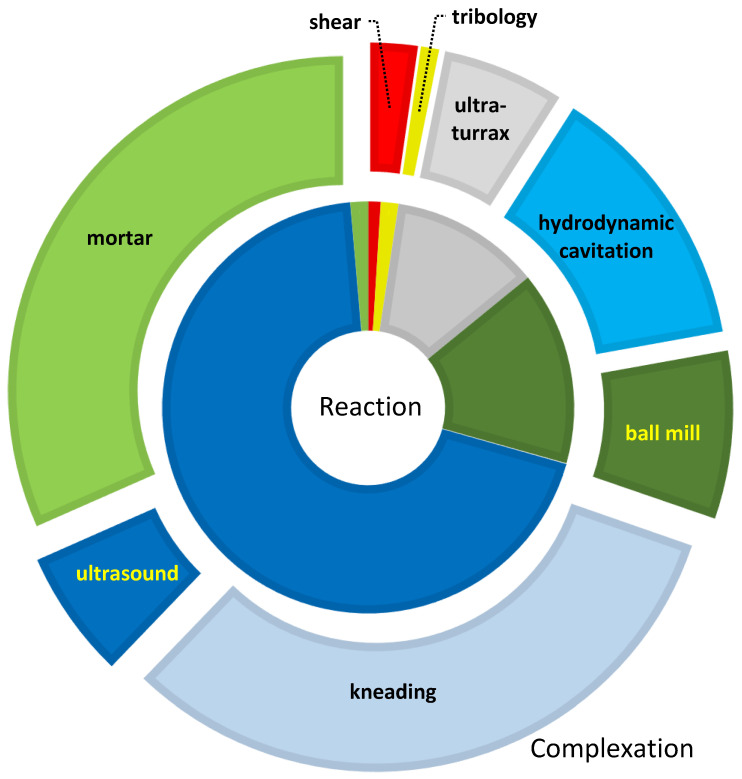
Participation of various mechanochemical methods in chemical transformations (inner slices) and complex preparations (outer arcs) in technical papers till 28 July 2021. Due to the overlaps of some topics, such as grinding and kneading, hydrodynamic cavitation/ultra-turrax, and ultrasonic mixing, the percentages carry little information.
